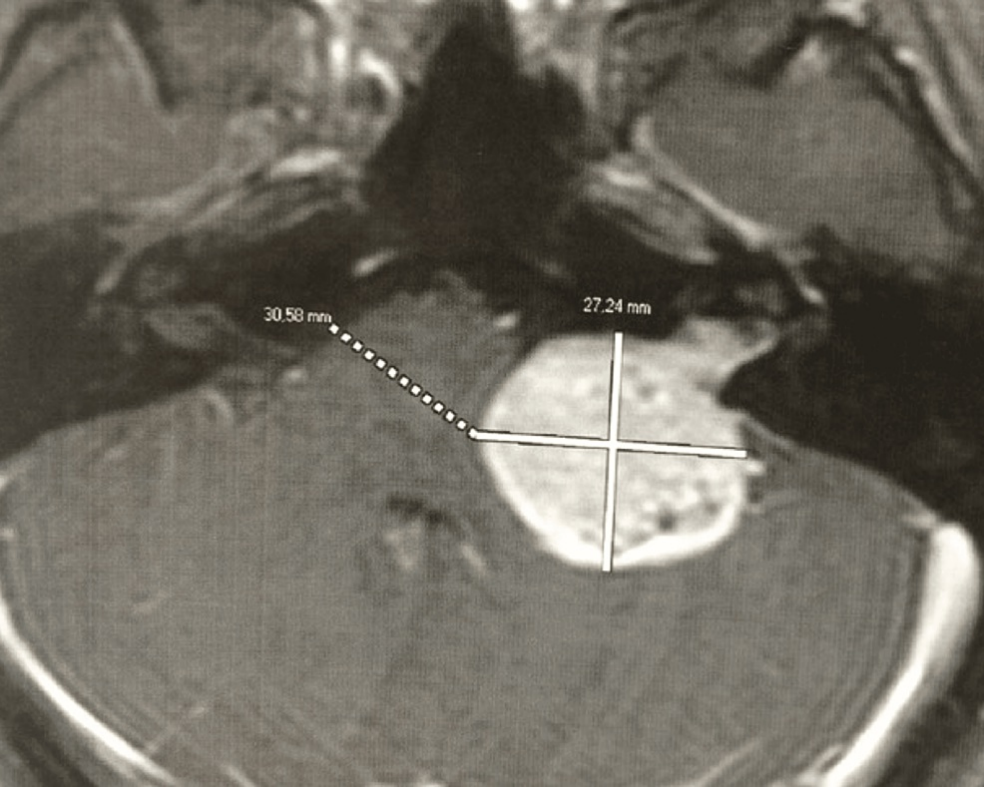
Axial contrast-enhanced T1-weighted MRI showed a large left-sided VS (two crossed white lines), grade T4b (Hannover classification), with severe brainstem compression
